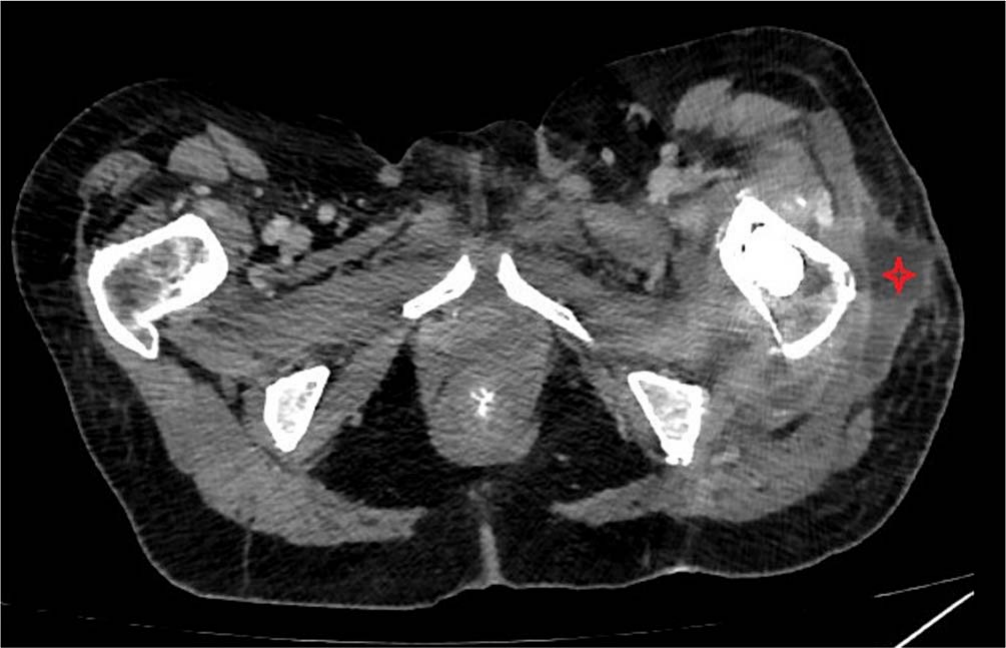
CT abdomen/pelvis showing subcutaneous collection at the upper lateral aspect of the thigh in the vicinity of the left proximal femur.
